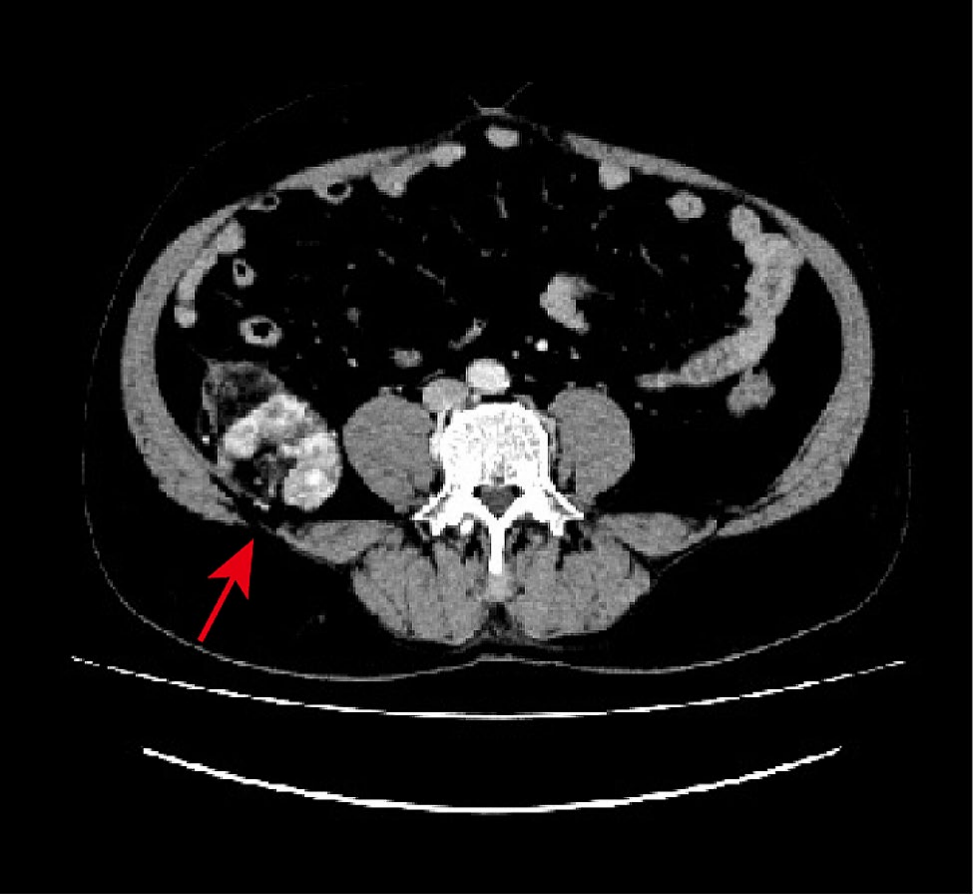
Contrast-enhanced computed tomography (CT) images clearly demonstrate a mass with predominantly fat attenuation adjacent to the right margin of the psoas major muscle in the retroperitoneum (red arrow). The mass exhibits irregular and nodular soft tissue, which displays significant enhancement on contrast-enhanced CT
